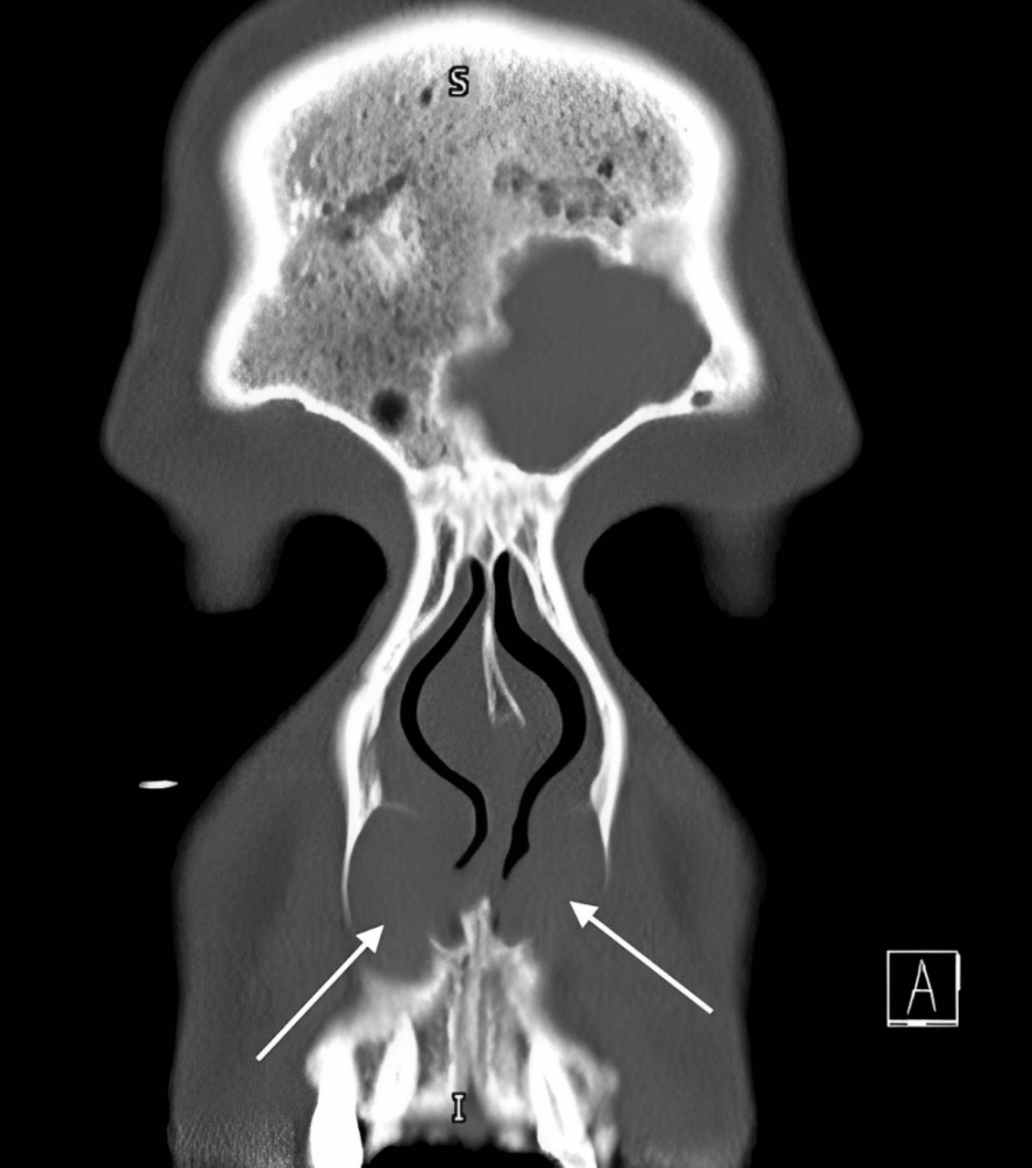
Coronal CT (soft-tissue window) of the anterior nasal cavity (performed on June 6, 2024) showing bilateral, well-circumscribed, low-attenuation submucosal lesions along the anterior nasal floor
